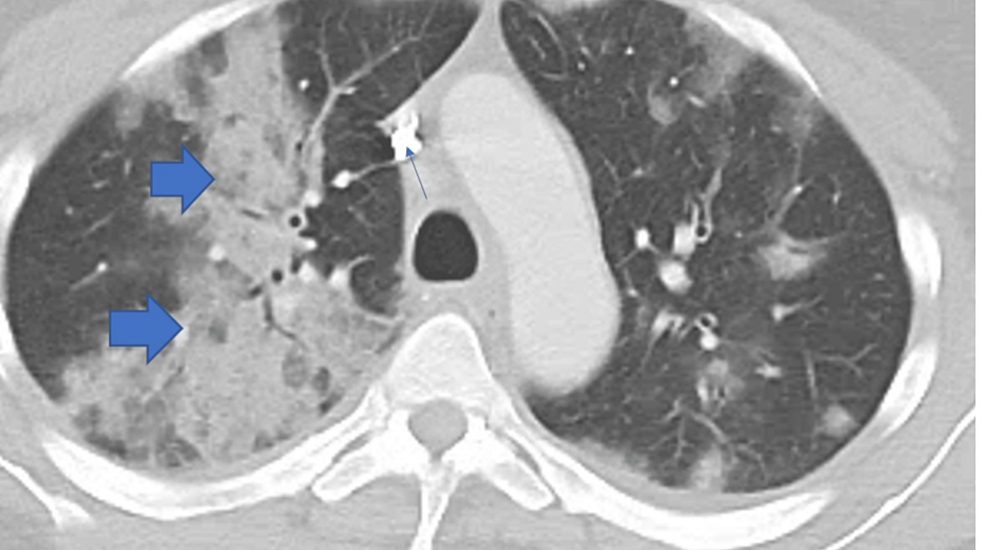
Computed tomography of the chest showing bilateral airspace disease (bold blue arrows on right-sided findings).
